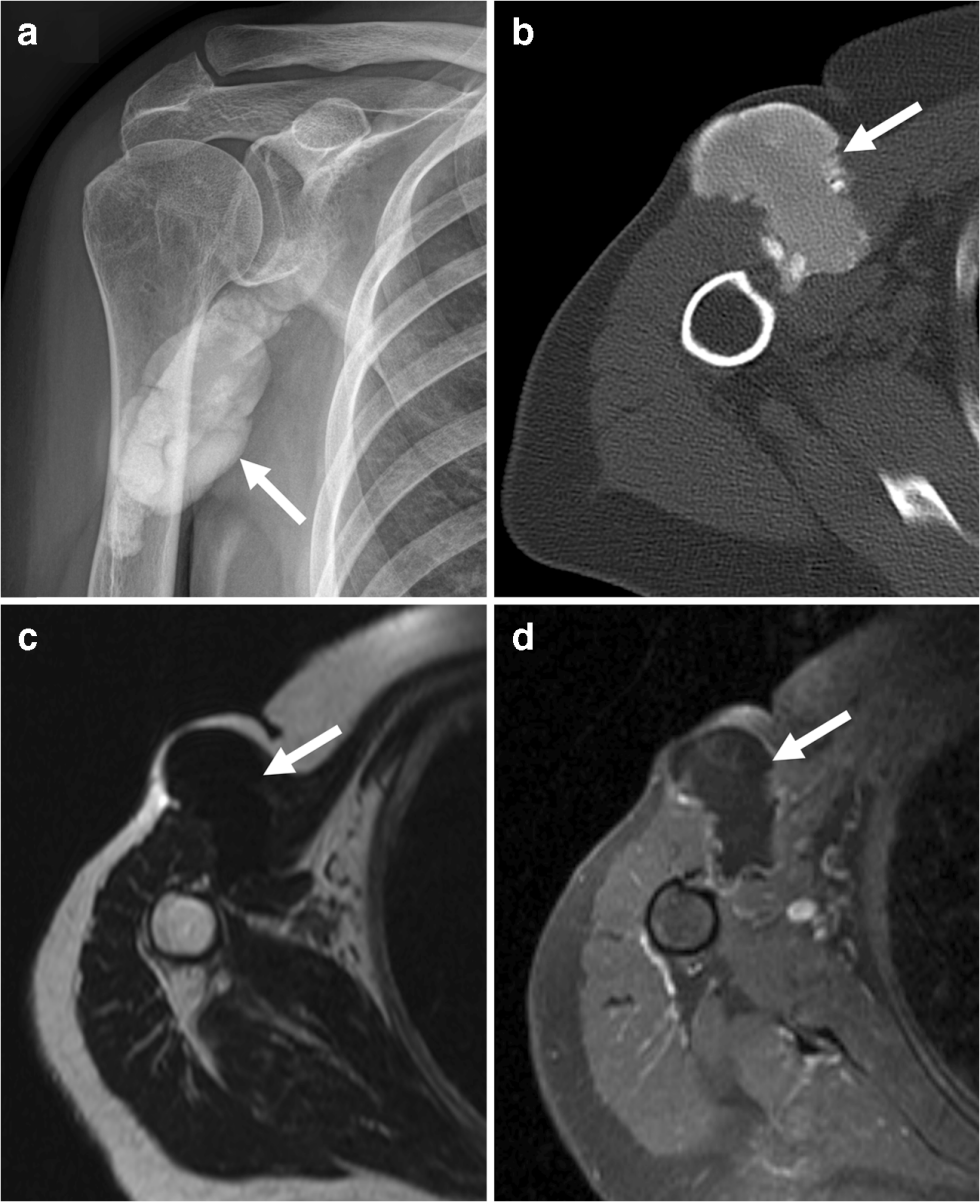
A 62-year-old woman with tumoral calcinosis. a Radiography shows a well-circumscribed calcified soft tissue mass (*arrow*) near the right shoulder. b Axial CT that the calcified mass is located within the lateral part of the pectoralis major muscle and extends subcutaneously. c Axial T2-weighted image shows that the lesion has low signal intensity. d Axial T1-weighted image with fat suppression demonstrates low signal intensity of the mass and a thin rim of peripheral enhancement
